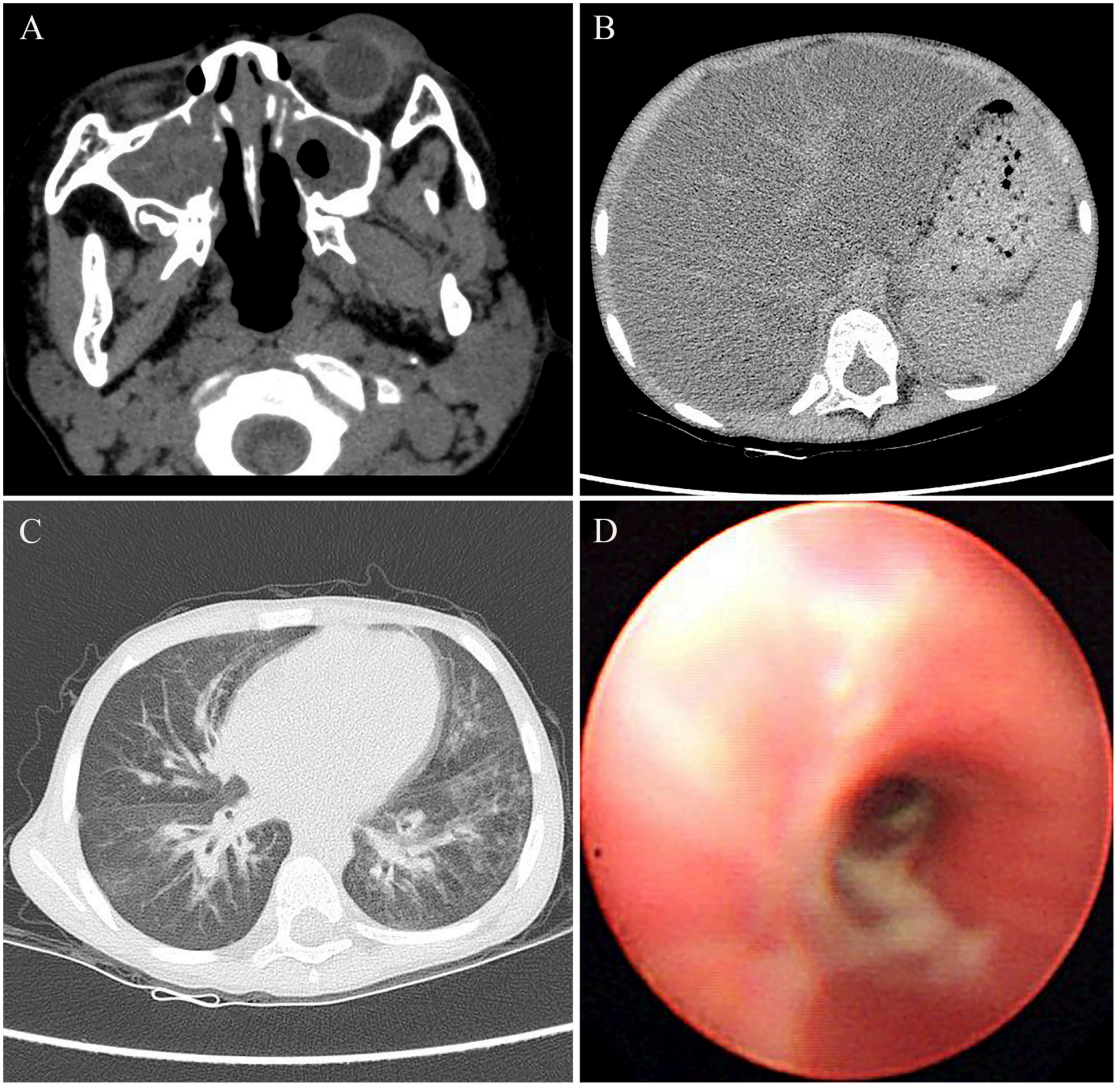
The images of CT and bronchoscopy in 2014, (A) maxillary sinuses were almost full of secretions, and (B) diffuse liver steatosis was observed. (C) Mild bilateral bronchiectasis was observed in CT, and (D) purulent secretions were observed in bronchoscopy.
